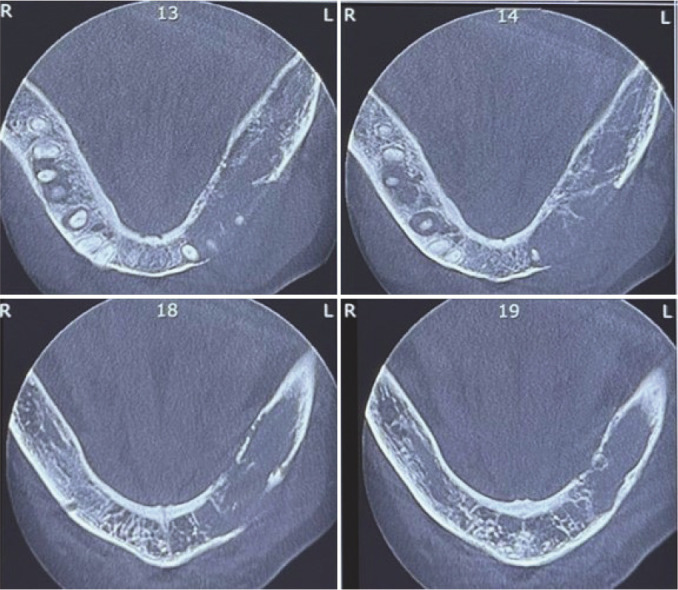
Axial views of the cone beam computed tomography reveal tumor extension and cortical destruction
